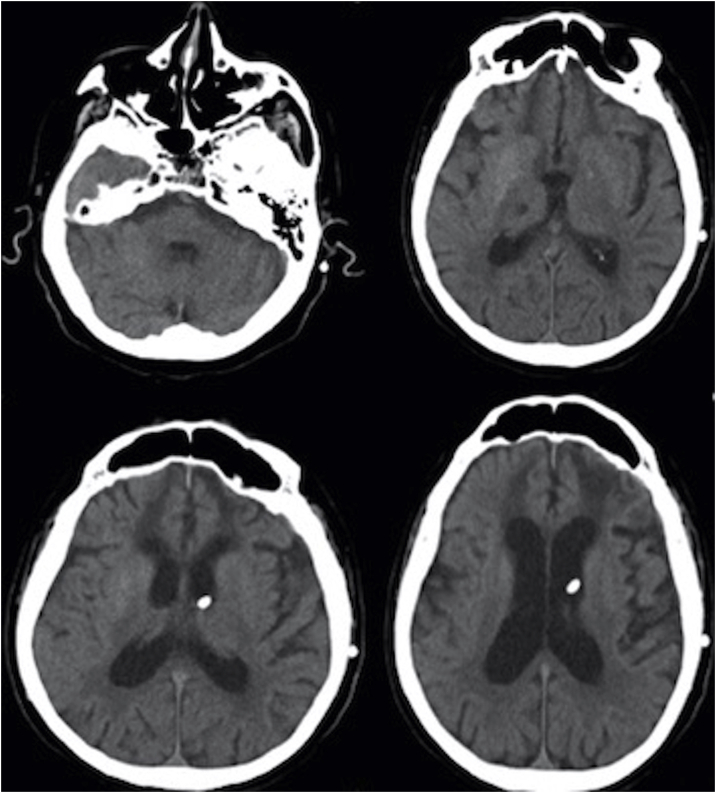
Cerebral Computed Tomography, axial view. Dilation of the ventricular system. Interstitial periventricular edema. Absence of blood density in the ventricular system.
